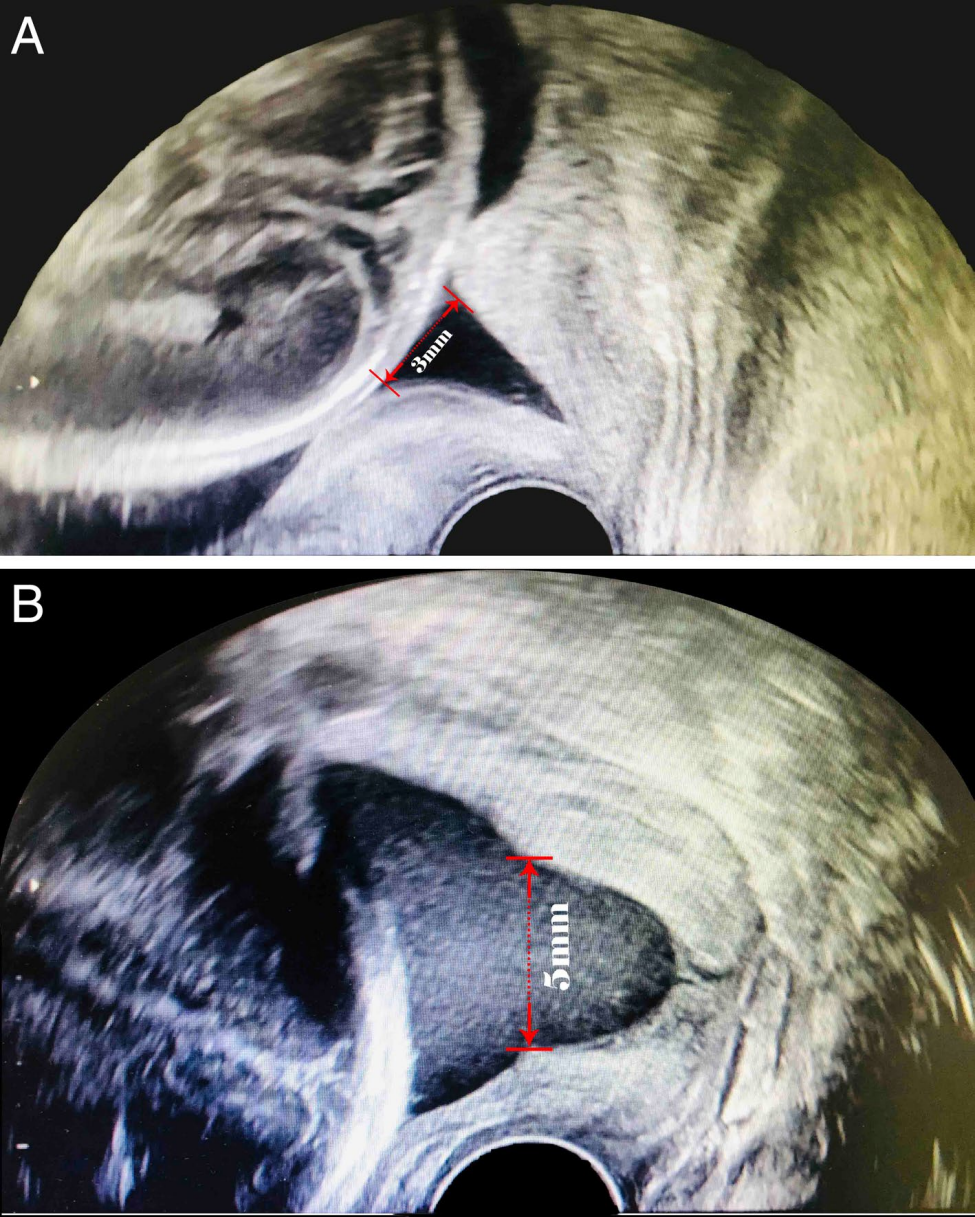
Cervical funneling detected by transvaginal ultrasound examination. (A) V-shaped funnel. (B) U-shaped funnel.
